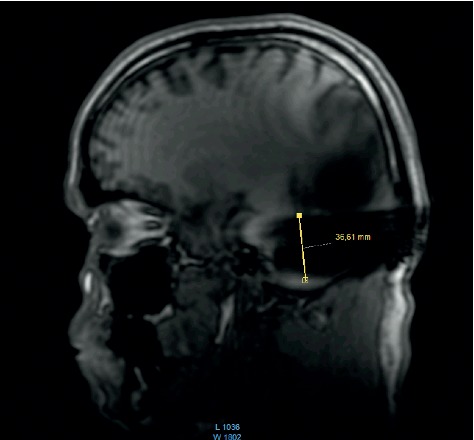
MRI pattern of the axial plain of a 3 T scanning Medel Synchrony Implant. White star indicates the hard artifact area. Circle indicates the soft artifact area.
